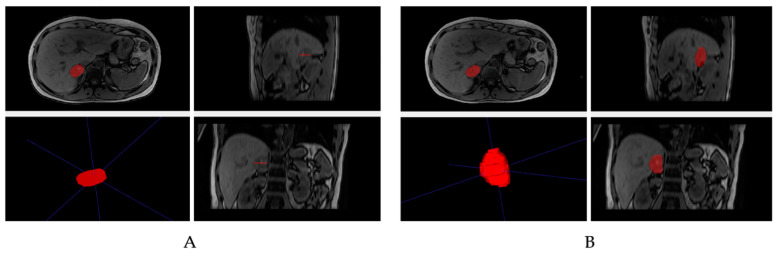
In this example, two-dimensional (A) and three-dimensional (B) approaches for adrenal lesion segmentation on magnetic resonance images are shown.
